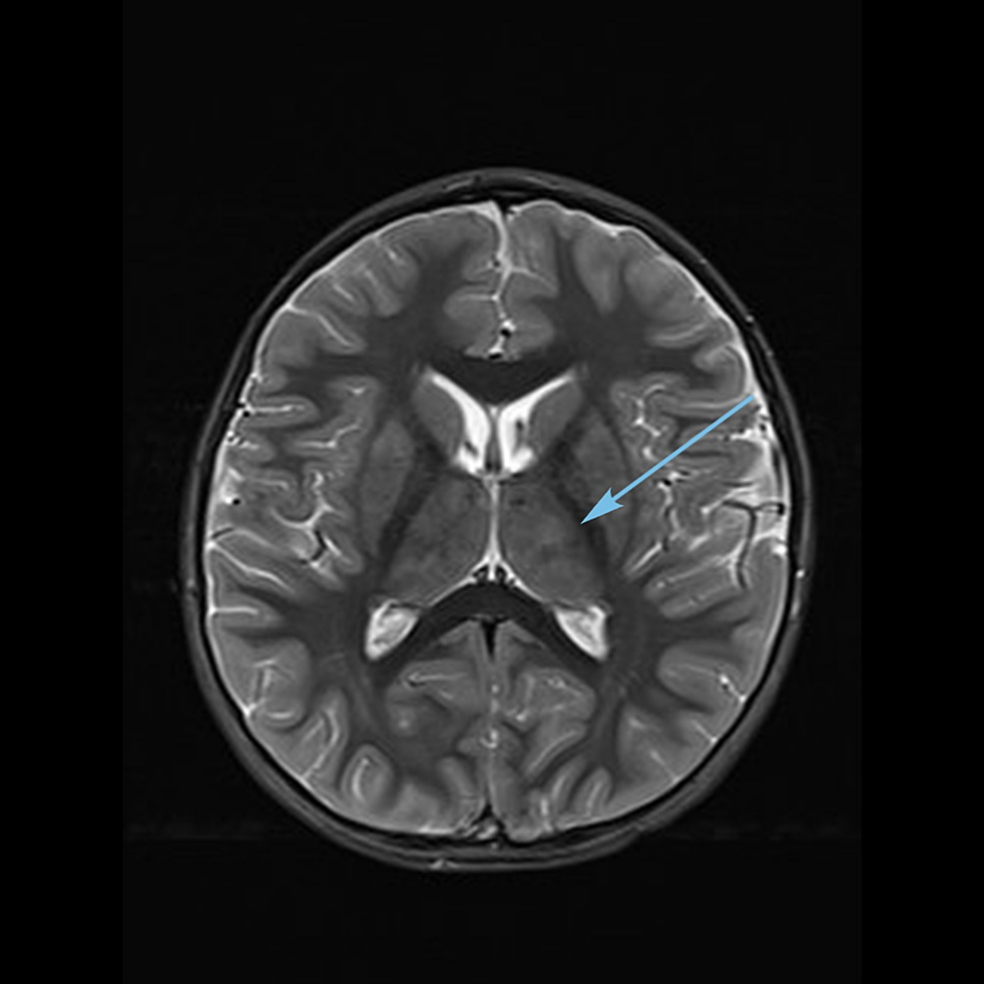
MRI brain T2 axial image showing thalamic hyperintensities (arrow).
